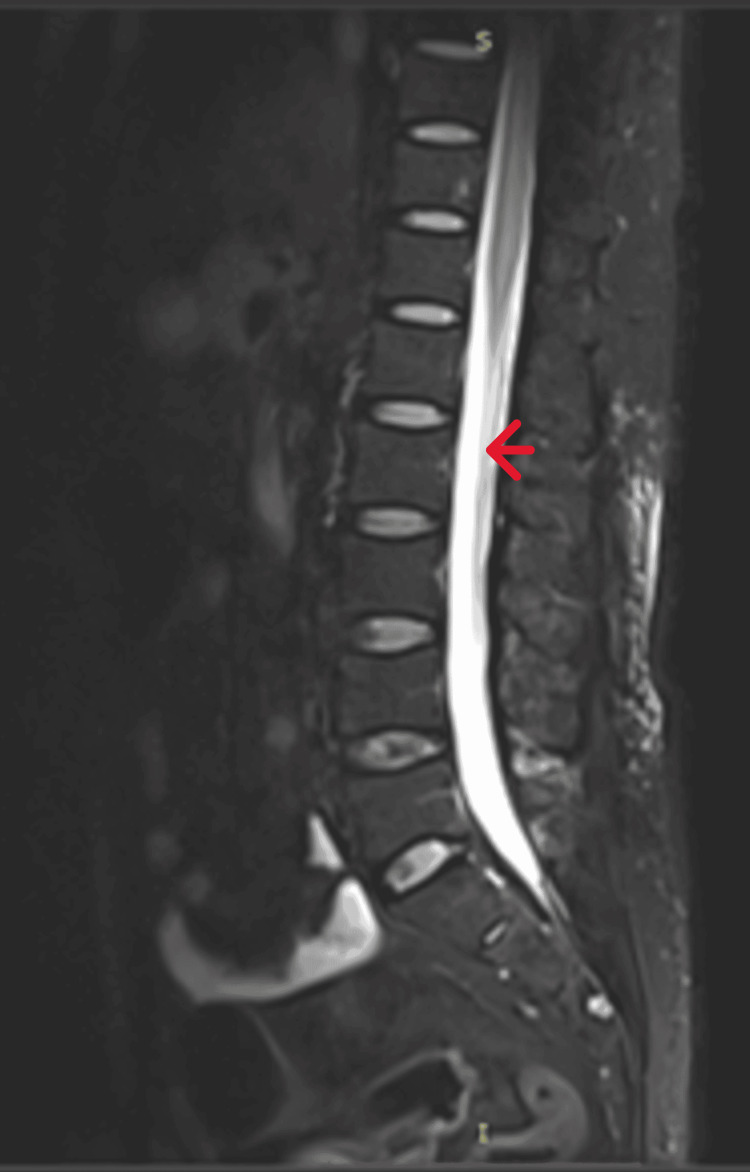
T2-weighted MRI of the lower thoracic, lumber, and sacral spine (sagittal section) showing long segment intramedullary high signal intensities extending up to the conus medullaris. MRI: magnetic resonance imaging
